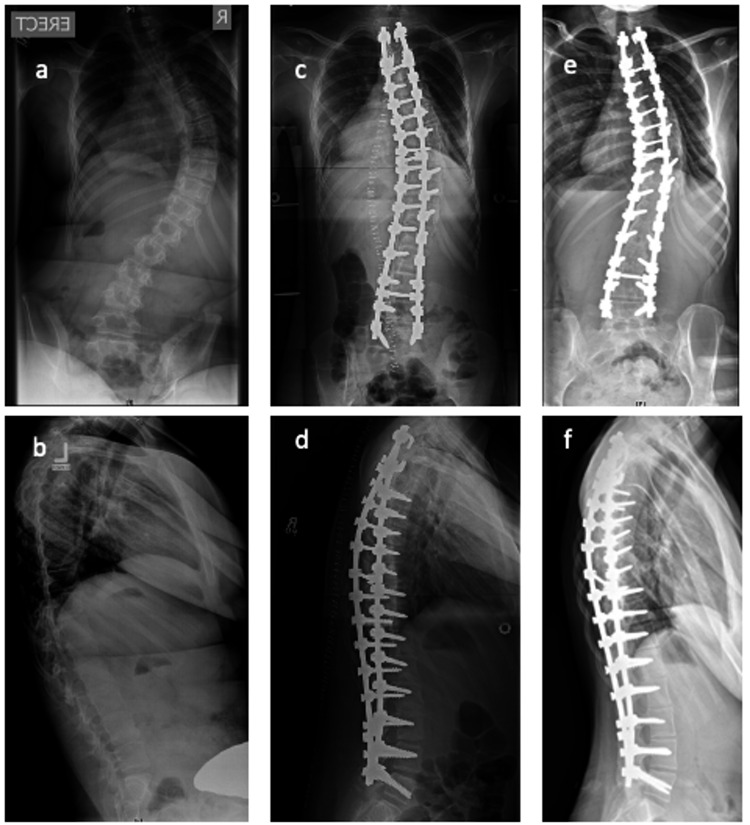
Plain radiographs of a 11-year-old female patient a) AP and b) lateral plain radiograph of an 11-year-old female patient with a coronal angular deformity of 79.5◦ and kyphosis of 70.7◦ and pelvic obliquity of 12.1◦. c) AP and d) lateral post-operative radiographs showing posterior instrumented fusion of T2 to L5 with a coronal angular deformity of 23.1◦, kyphosis of 43.5◦ and pelvic obliquity of 1◦. This correction is maintained at five years in e) AP and f) lateral radiographs.
